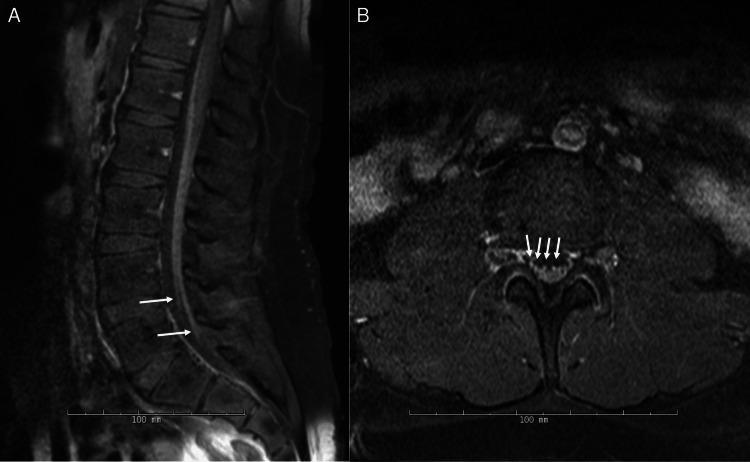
MRI of the lumbar spine from the second admission Image A (left): Postcontrast T1-weighted sagittal view demonstrating diffuse smooth enhancement of the cauda equina. Image B (right): Postcontrast T1-weighted axial view demonstrating enhancement of the cauda equina nerve roots.
